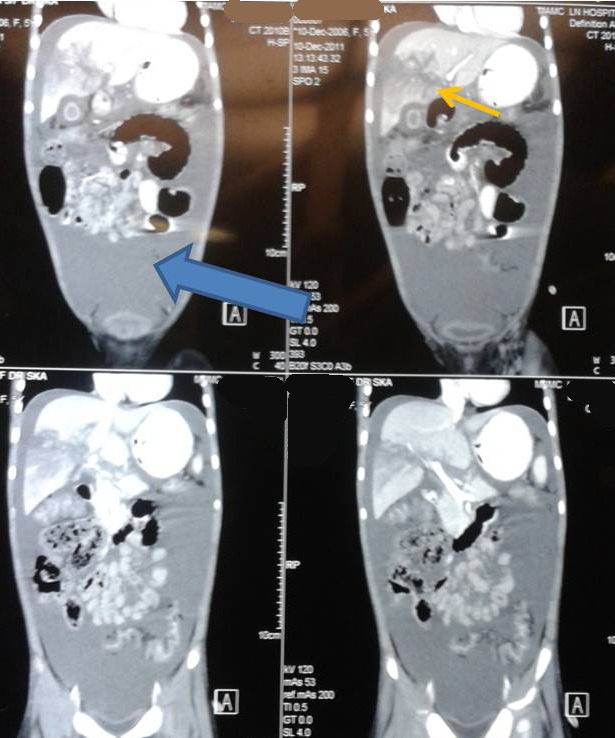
Figure 1:CT abdomen showing hypoechoic hepatic laceration (marked with thin arrow) with fluid collection (marked with thick arrow) in peritoneal cavity.
